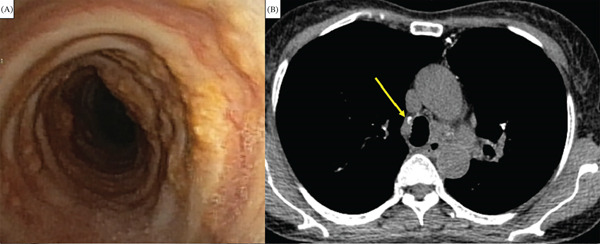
Tracheobronchial amyloidosis. (A) Bronchoscopic view showing irregular mucosal infiltration and luminal narrowing. (B) Axial chest CT (mediastinal window) demonstrates diffuse tracheal wall thickening accompanied by punctate calcification (yellow arrowhead).
